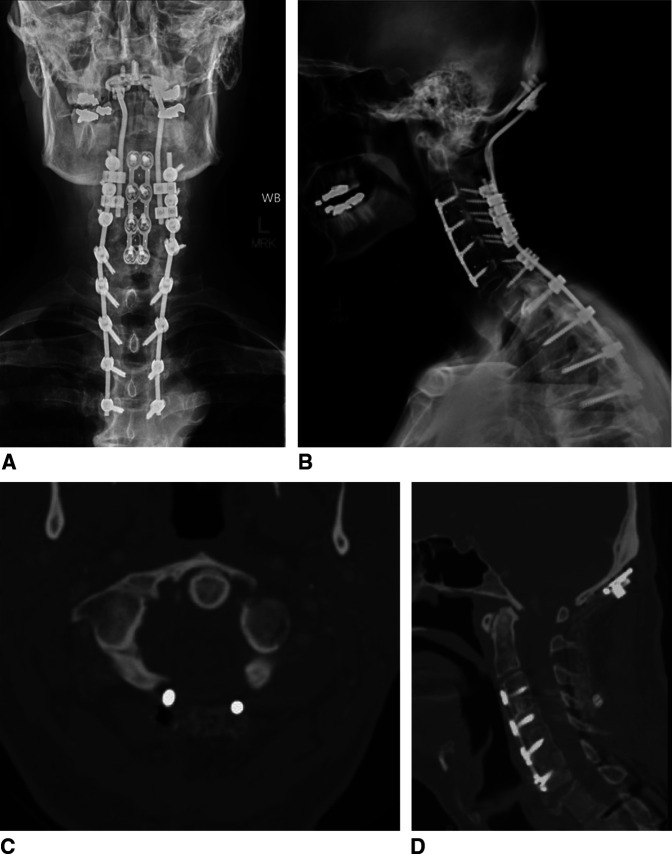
Radiograph demonstrating A, AP and (B) Lateral upright postoperative cervical x-rays after occipitocervical fusion with extension to previous instrumentation and fusion with improved alignment. C, Axial and (D) Sagittal images obtained from the patient's postoperative CT angiography demonstrates the known C1 fracture nonunion with no significant change in the cranial settling or posterior displacement of the occiput.
